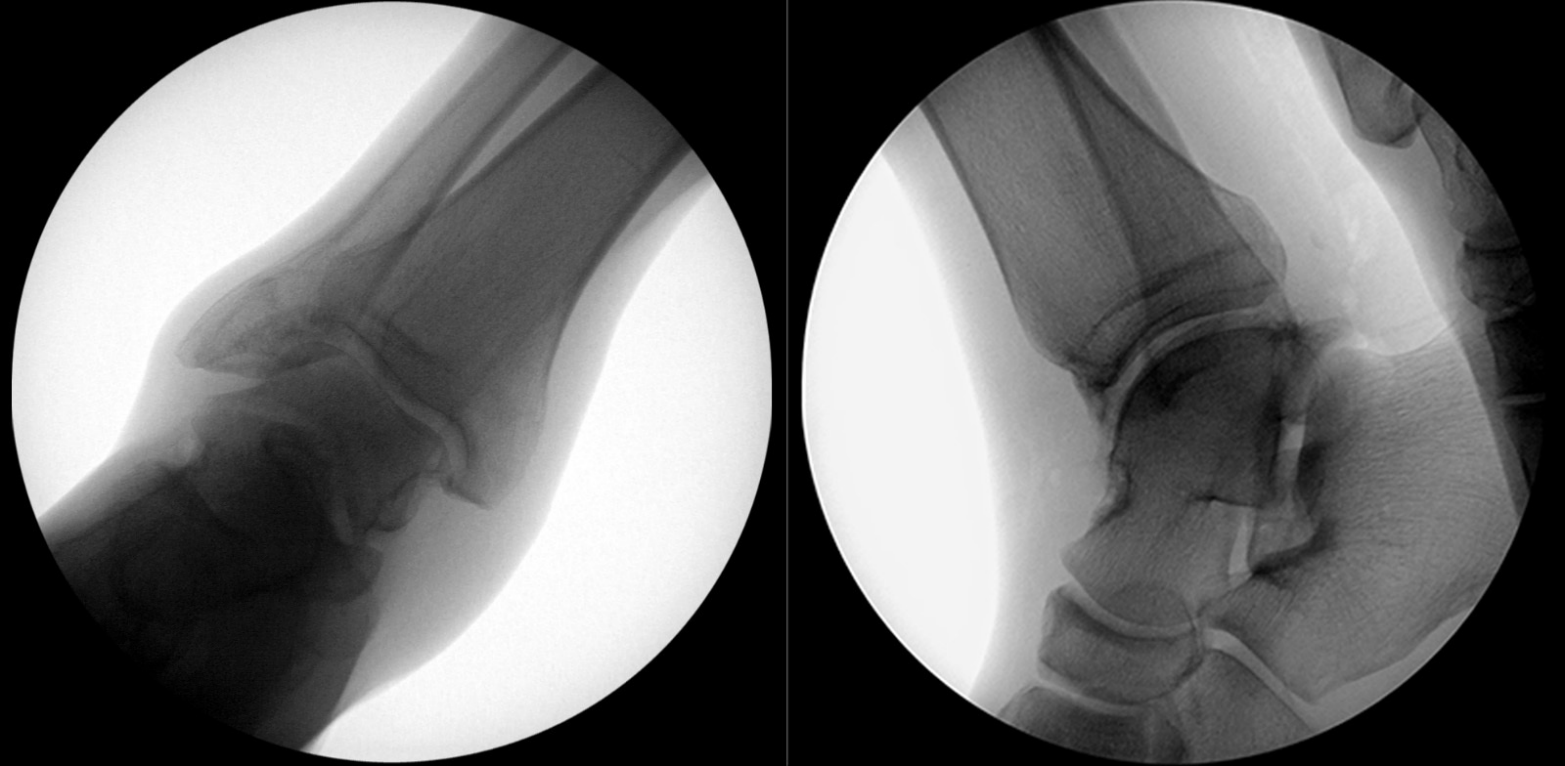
Intraoperative Fluoroscopic Imaging. Removal of all loose bodies was verified by intraoperative fluoroscopic imaging. Anteroposterior radiograph shown on the left, and lateral radiograph shown on the right.
